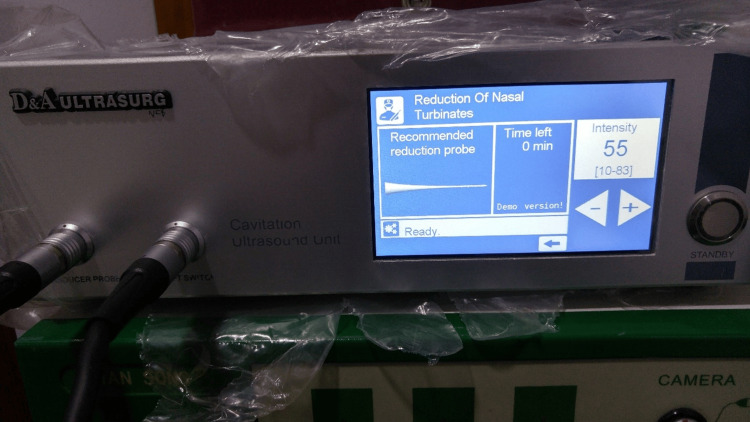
D & A Ultrasurg ultrasound device allows the adjustment of intensity via a touch monitor The intensity used in the conducted study was 55.
